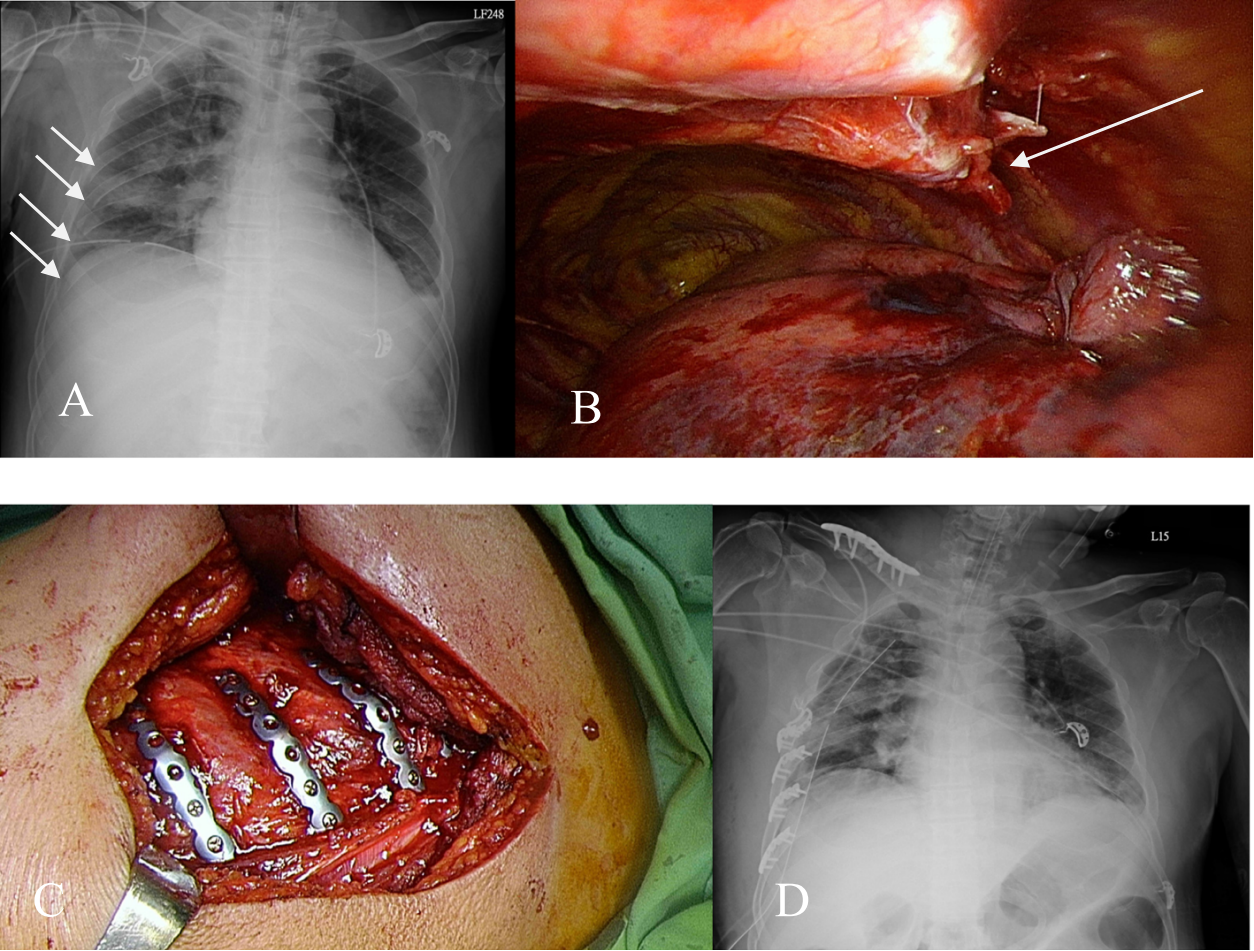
a Right side 5th to 8th rib bicortical fractures (white arrow) along with lung contusions. b VATS evacuation of hemothorax combined with localization of fractured rib (white arrow). c Fixation of fractured ribs using titanium plates with locking screws on the outer surface of ribs. d Chest X-ray after the operation
